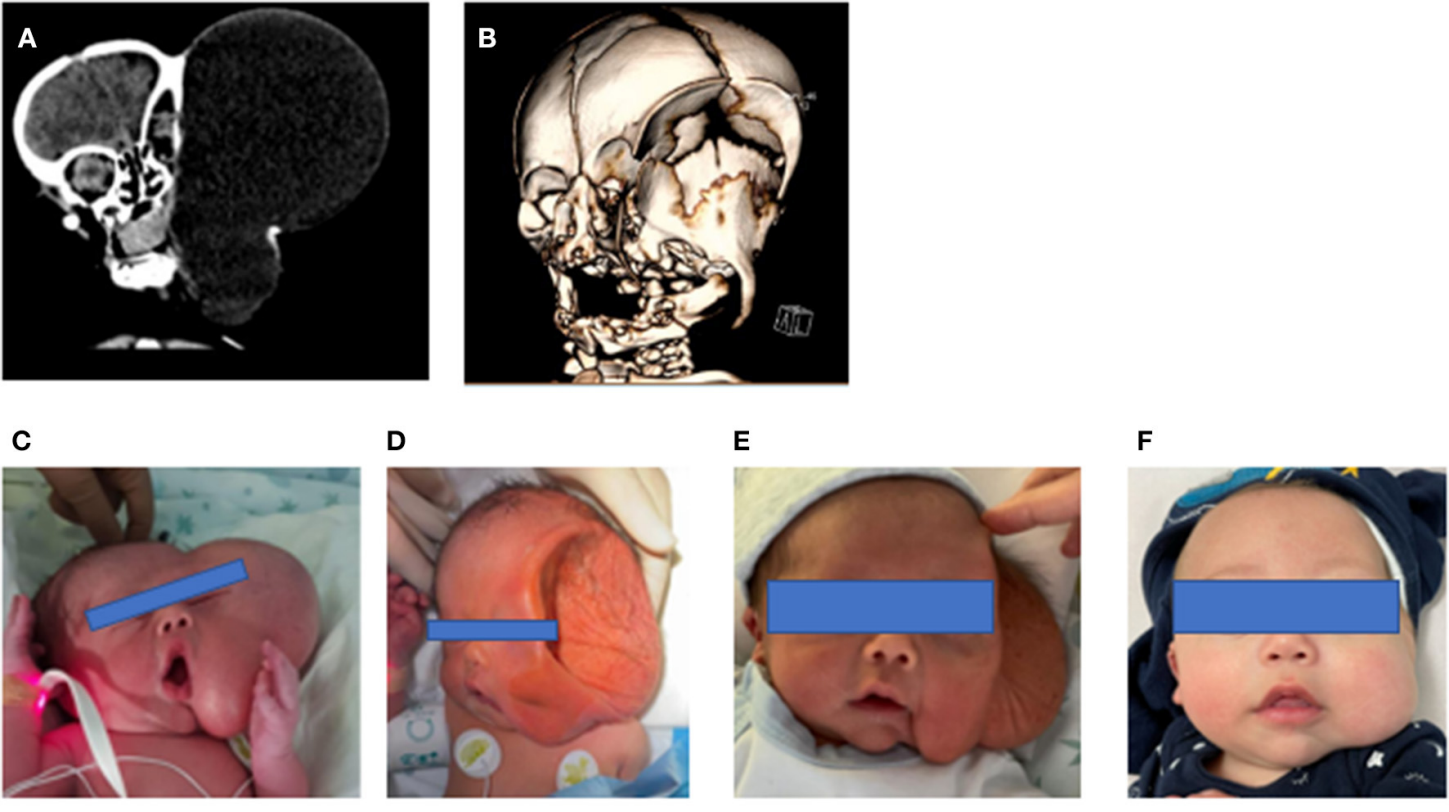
(A) Postnatal TC scan, (B) 3D CT reconstruction, (C) pre-sclerotherapy appearance, (D–F) post-sclerotherapy evolution.
